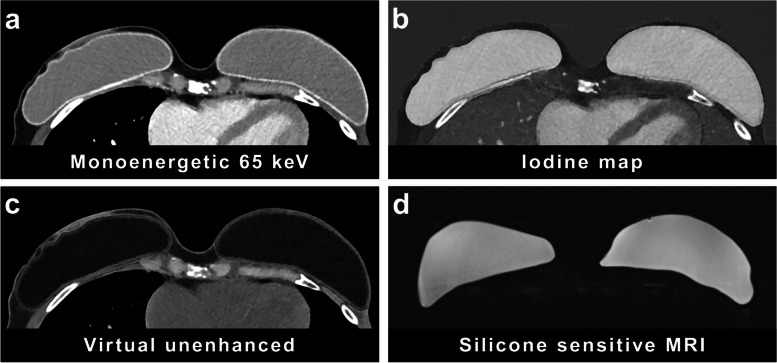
Photon-counting computed tomography images (a–c) and magnetic resonance imaging images with silicone-sensitive sequences (d) of two intact subpectoral breast implants on both sides. Axial monoenergetic 65-keV reconstruction (a) and material decomposition reconstructions (iodine map [b] and virtual unenhanced [c]) show distinct contrast properties of the implants with high density of the silicone in the iodine map with window level 40 HU and window width 400 HU (b)
